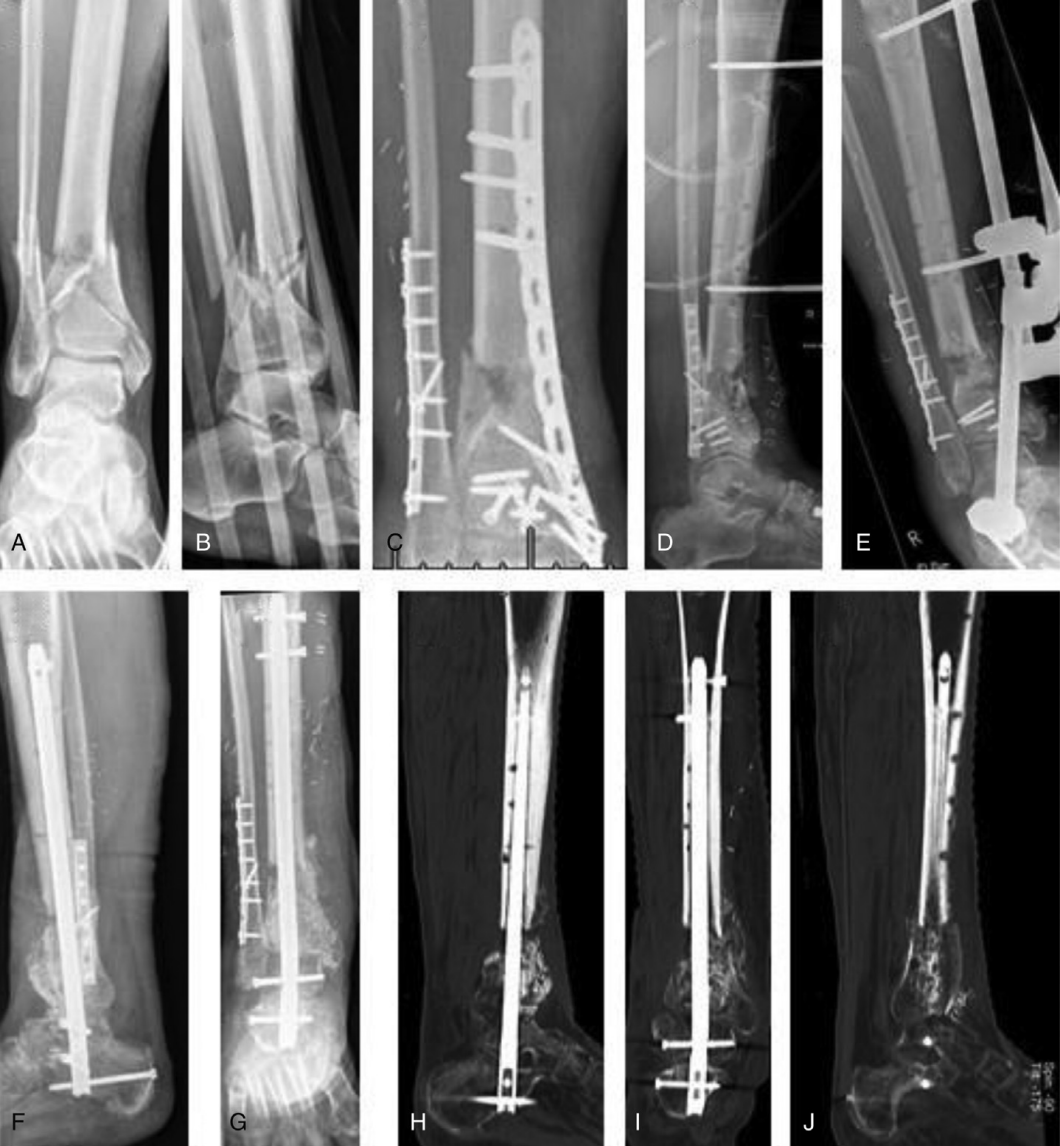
Fifty-nine-year-old male with chronic osteomyelitis after pilon fracture (A, B). Initial treatment with AO external fixator followed by plate osteosynthesis. Ten months after initial treatment, persisting secretion from wound and failure of osteosynthesis was observed (C, D, E). Treatment with debridement, arthrodesis, and bioglass (F, G). Follow-up CT scans show partial consolidation (H, I, J), the patient was pain free and able to bear full weight. CT = computed tomography.
